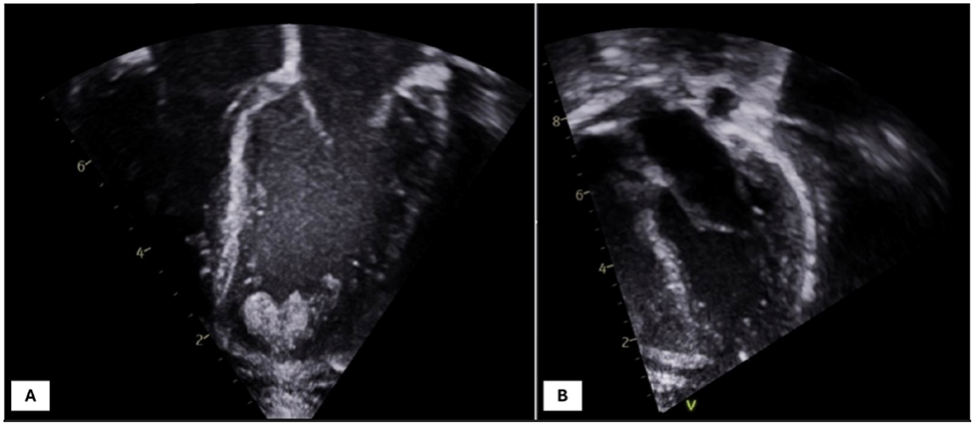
Echocardiogram of a 12-month-old male showing thrombus. A: Thrombus in the left ventricle apex measuring 6x7 mm and 6x6 mm, bilobed or two adjacent clots. Small to moderate pericardial effusion. B: Resolution of left ventricle thrombus.
